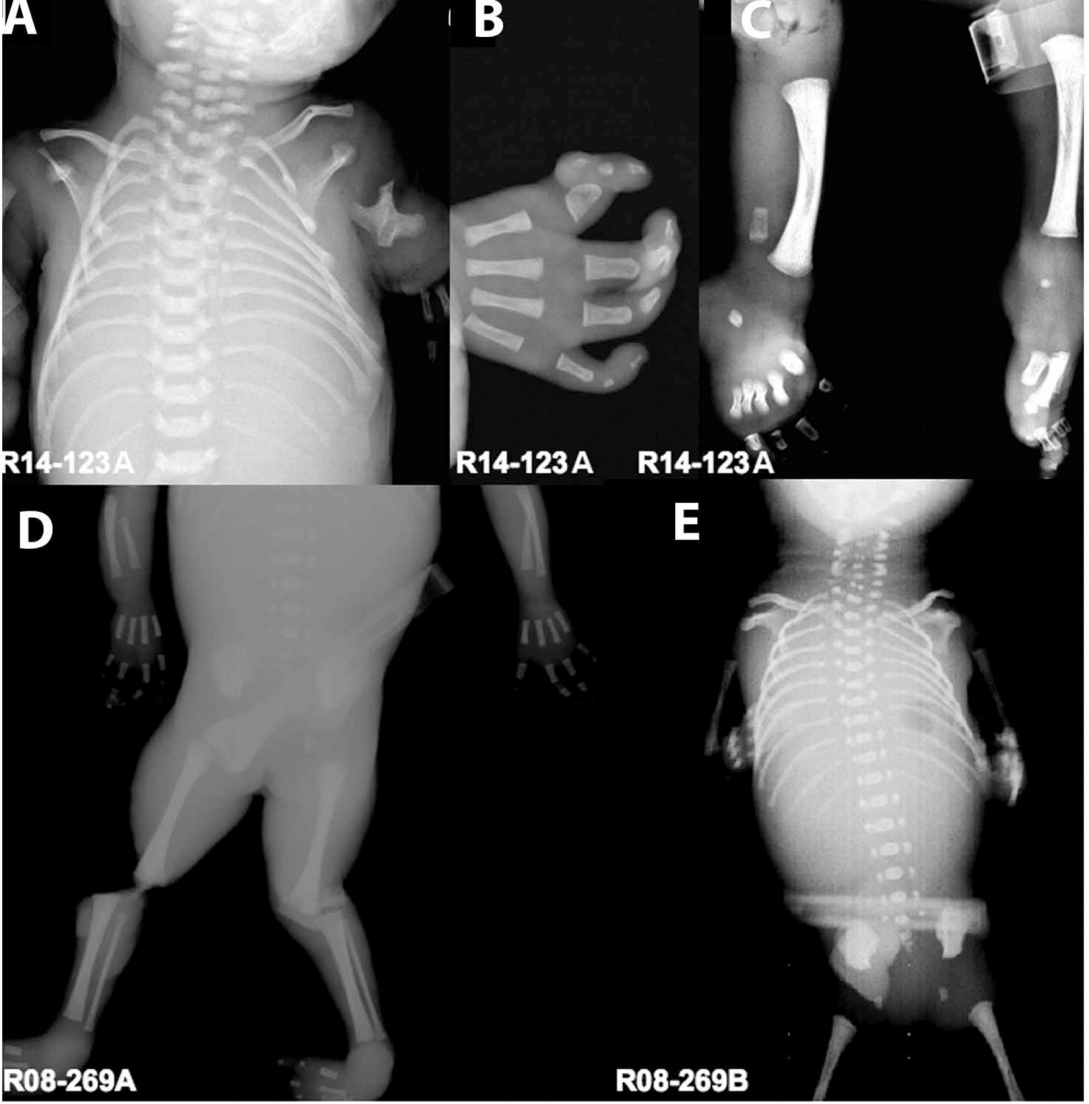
Radiographic phenotypes of cases R14-123A and R08-269A & B. (A) A/P radiograph of the chest of R14-123^a^ showing small scapulae, 11 ribs, and abnormally formed hypoplastic humeri with radioulnar synostosis. (B) Hand radiograph showing oligodactyly, hypoplastic carpal bones and preaxial polydactyly. (C) Bilateral lower extremities showing hypoplastic or absent fibulae with small stippled calcanei. (D) A/P radiograph of R08-269A showing hypoplastic radii, oligodactyly, absent thumbs, thin fibulae, and club foot. (E) A/P radiograph of R08-269B showing 11 ribs, absent radii and ulnae.
